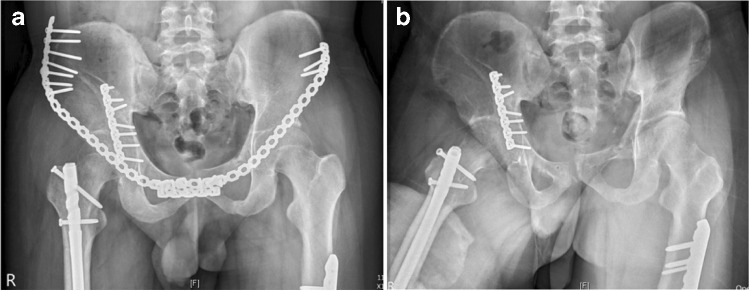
a Post-operative radiographs of a 28-year-old man (no. 9) showing right iliac wing fracture, right acetabular both column fracture, left superior and inferior pubic rami fracture, pubic symphysis diastasis, and bilateral femoral open fracture showing anatomical reduction. b AP radiographs after removal of SAPB showing excellent radiological outcome with excellent functional outcome
